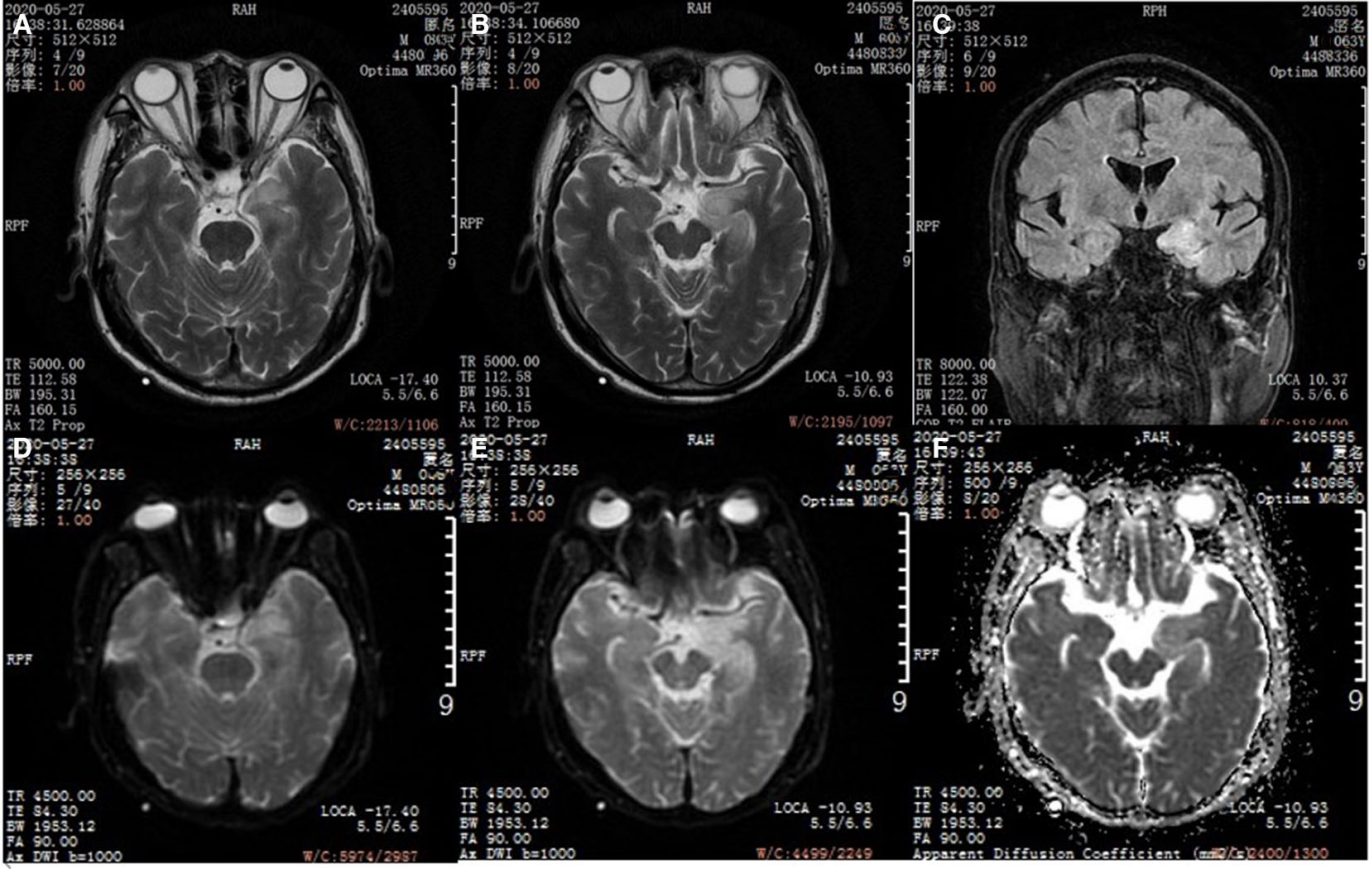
Head magnetic resonance imaging (MRI). (A,B) T2WI axial image in a patient with anti-GABA B encephalitis. (C) FLAIR corona scanning. (D,E) DWI axial image. (F) ADC axial image.
